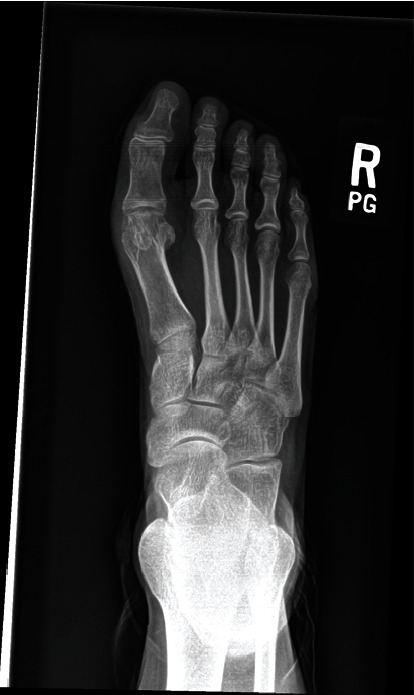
Anteroposterior radiograph of the right foot taken three years post-OCA implantation with complete resolution of hallux rigidus.
